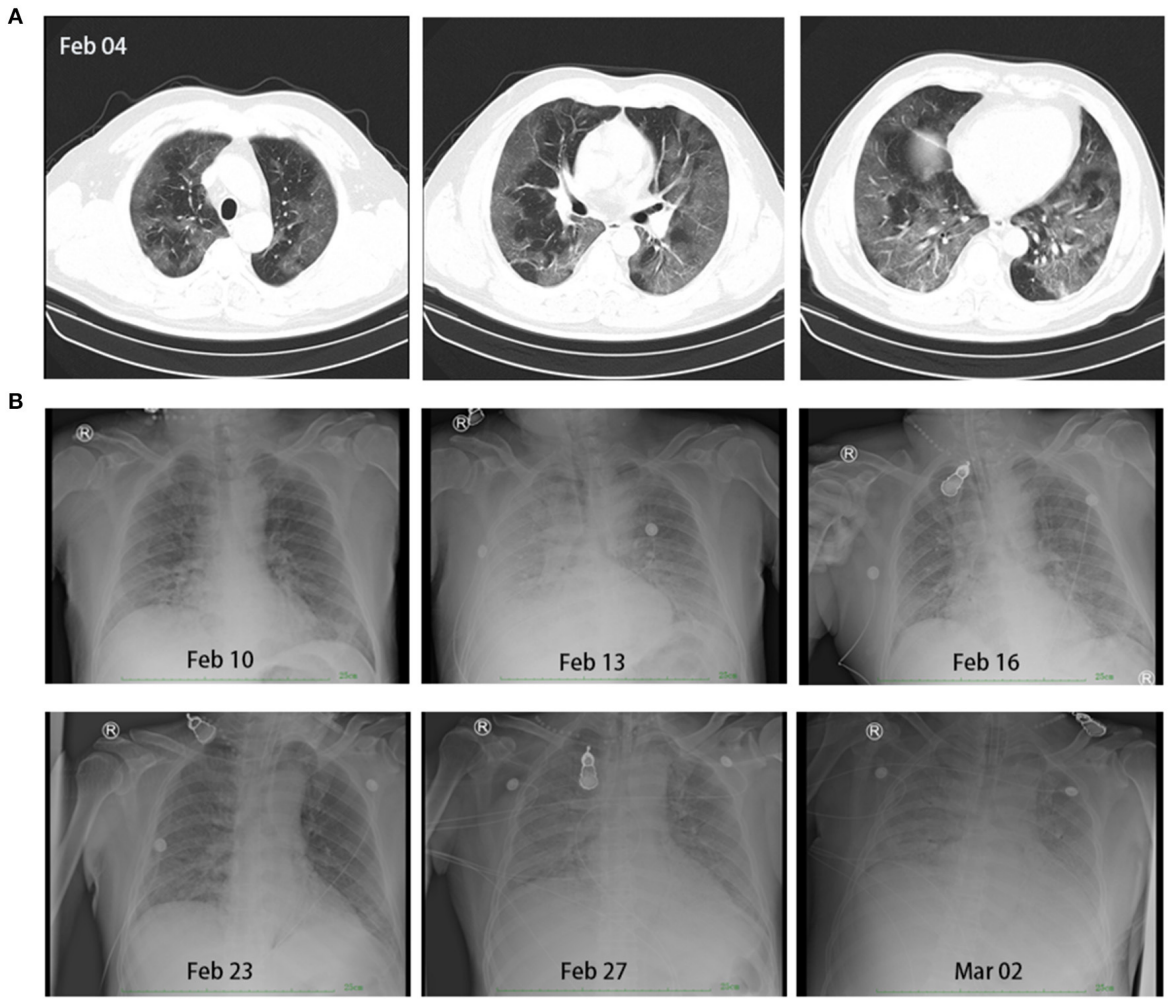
Chest-computed tomography of the patient on February 4, 2020, showed signs of infection indicating the possibility of viral pneumonia (A). Continuous chest digital radiography during treatment from February 10 to March 2 (B).
